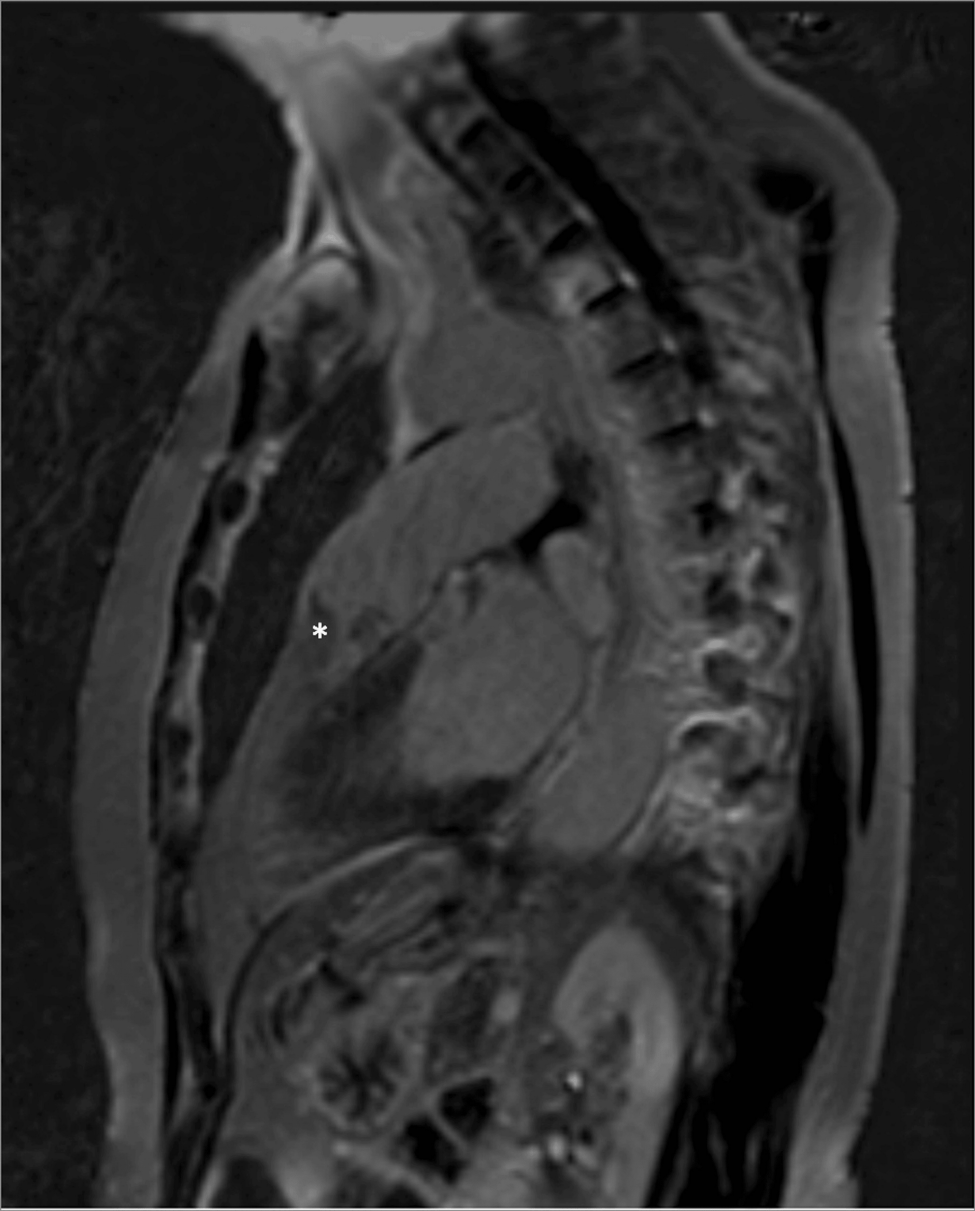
Cardiac Magnetic Resonance Late Gadolinium Enhancement Preoperative cardiac magnetic resonance imaging with late gadolinium enhancement demonstrating a pulmonary valve mass without significant contrast uptake (*).
